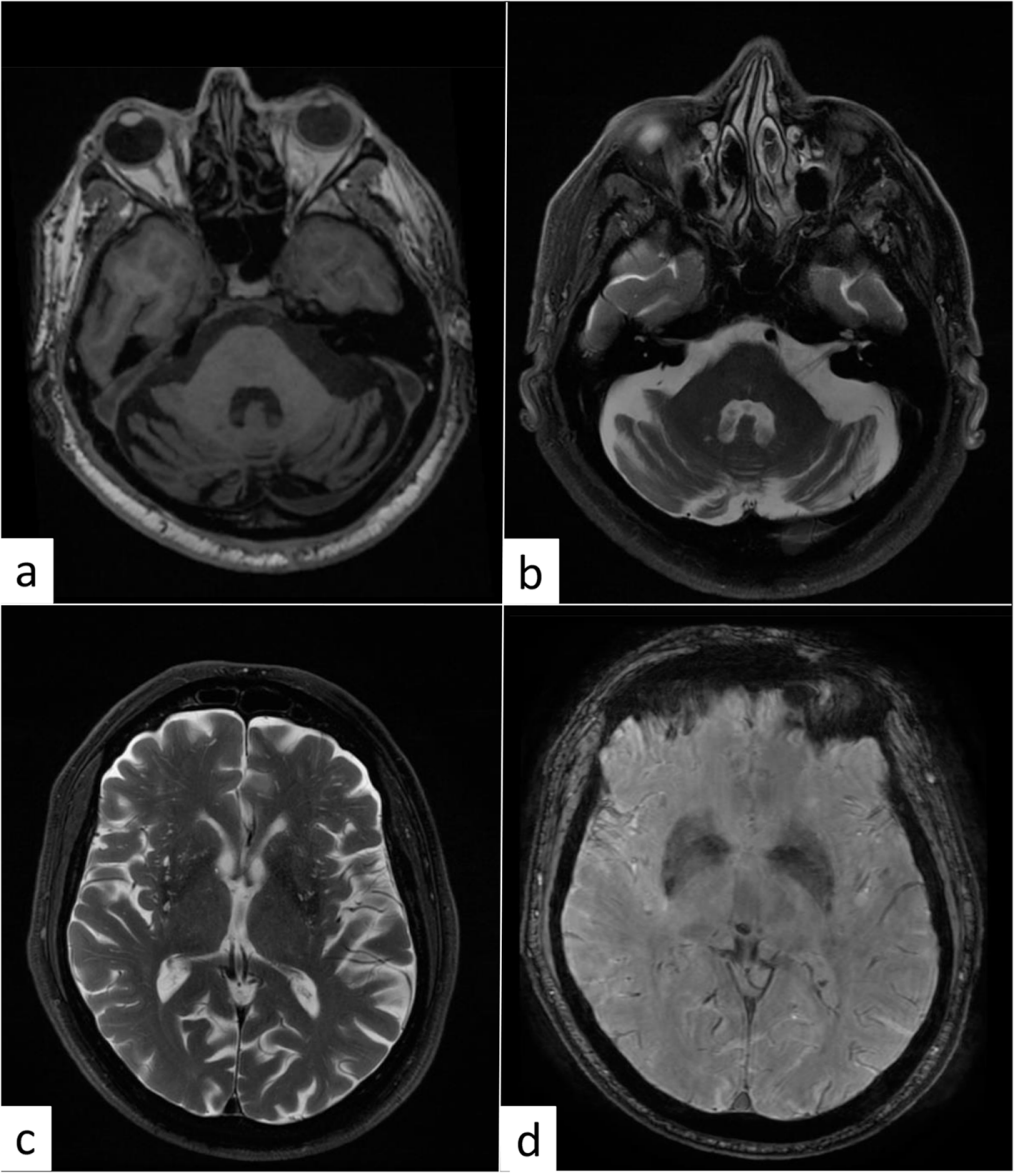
a T1 axial MRI scan shows bilateral hemispheric cerebellar atrophy b T2 axial MRI scan shows bilateral hemispheric cerebellar atrophy. c T2 MRI scan shows mild diffuse atrophy and enlarged perivascular space. d SWI MRI scan at the striatum level
